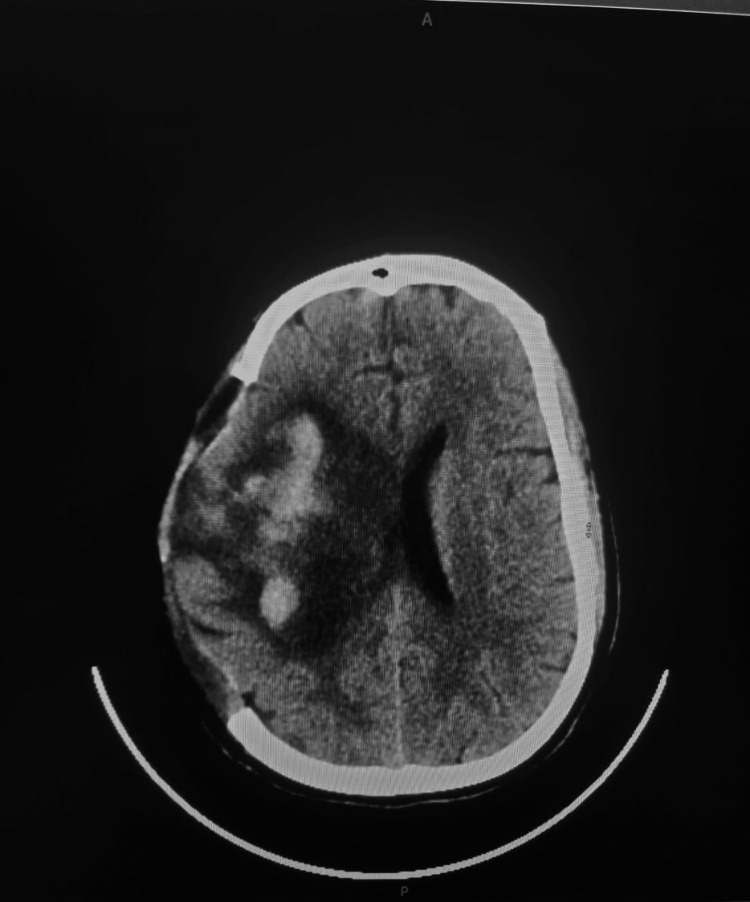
Follow-up CT of the brain on day 8 post-craniectomy
